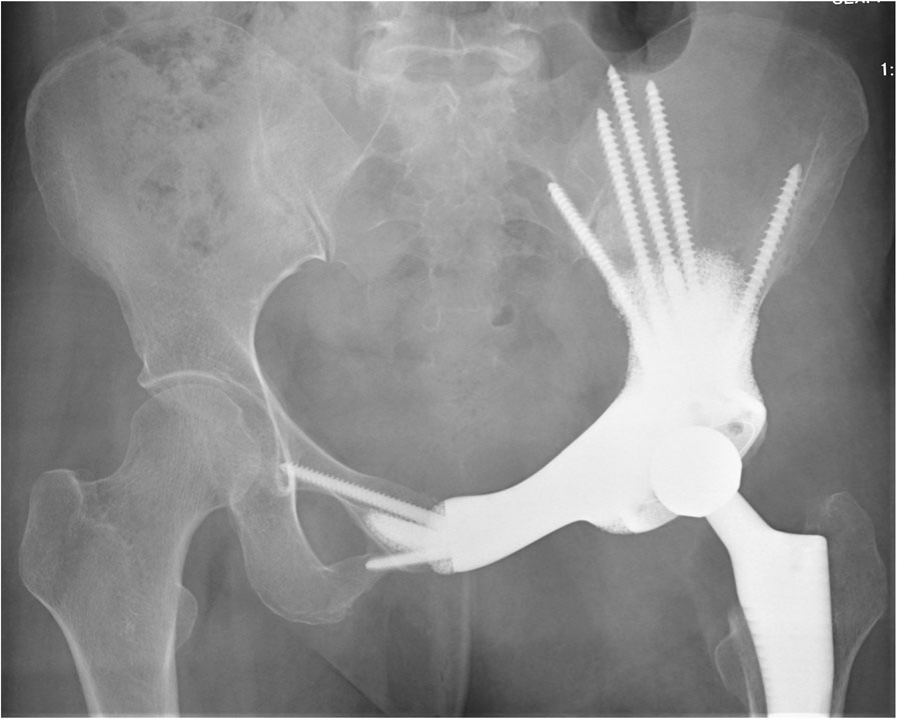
Two months after the operation, plain radiograph showed well alignment of endoprosthesis. Five screws were inserted to the ilium, and two screws were inserted to the contralateral pubis
